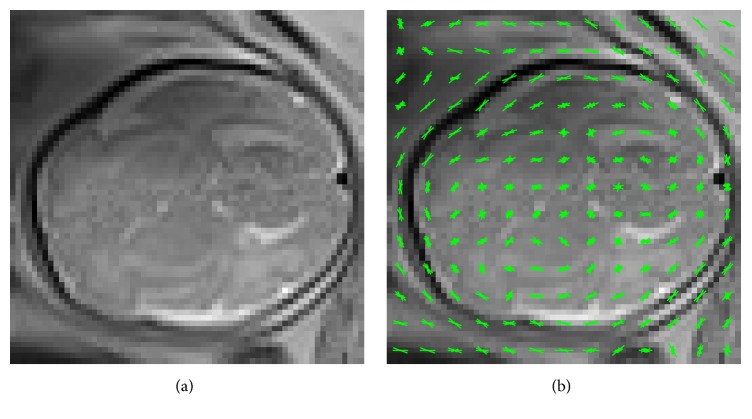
An example of an axial fetal MR image used from the training database (a) and the resulting computed HOG descriptor overlaid on the example image (b).
